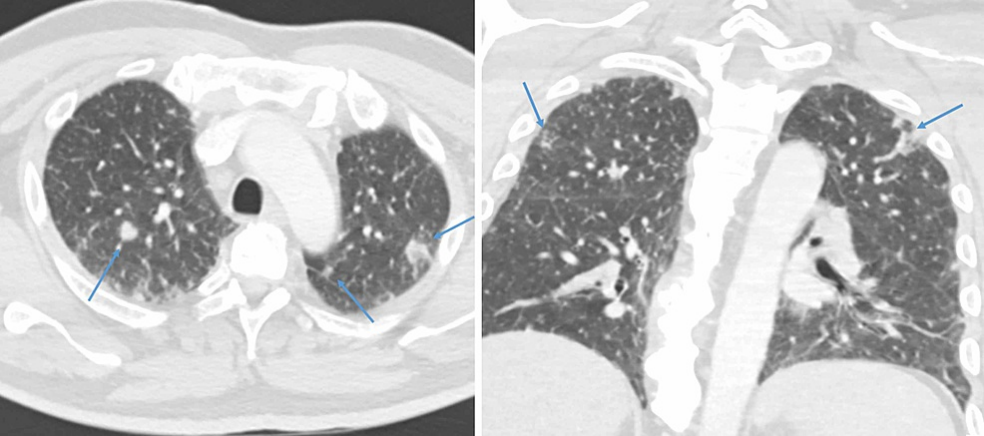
Axial and coronal CT chest images demonstrating numerous pulmonary nodules
